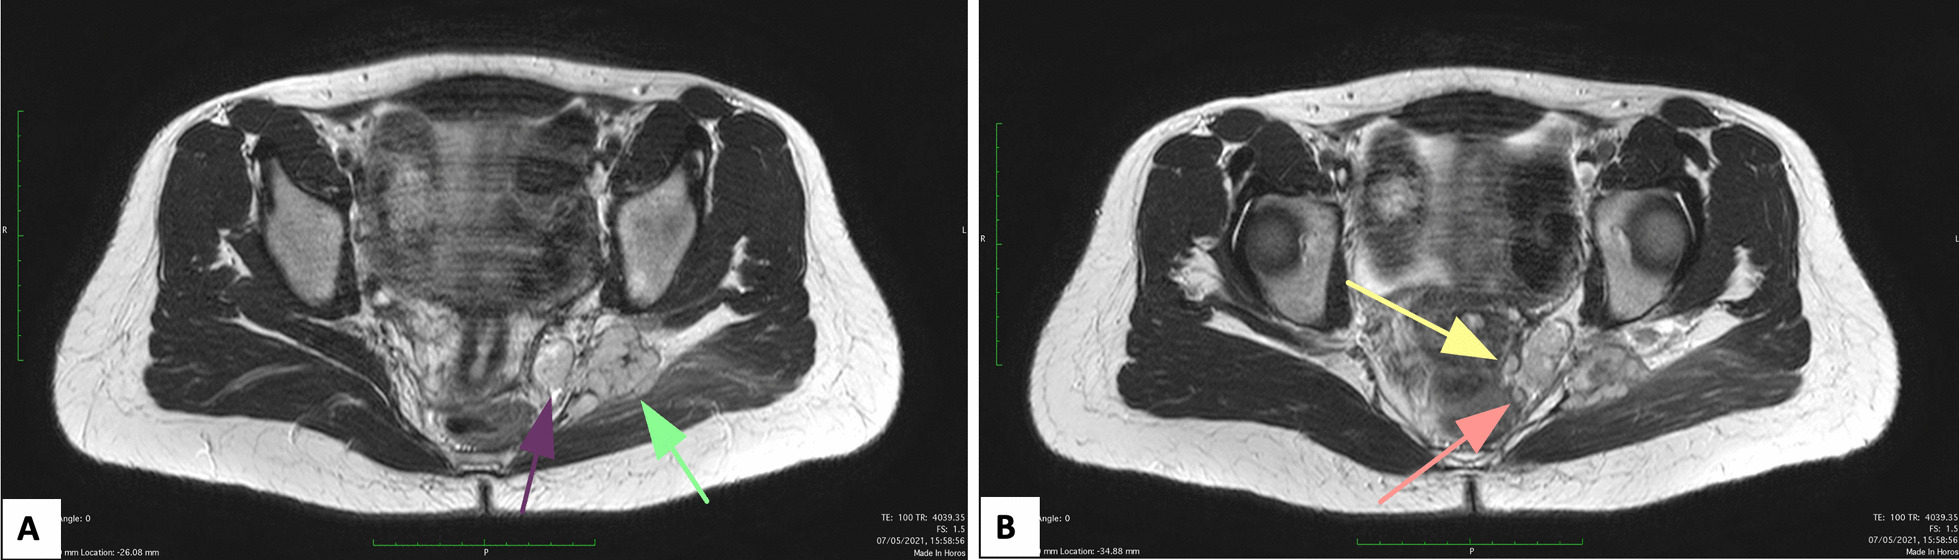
Magnetic Resonance Imaging (MRI) axial T1 weighted sequencies of the pelvis showing multiple nodules: A irregular nodule in gluteal region between the large gluteal muscle and the piriformis muscle (green arrow). Double nodule at the apex of ischiorectal fossa which is contiguous to the largest gluteal nodule (purple arrow); B two little nodules in the left obturator space, next to distal anal canal (yellow and pink arrow)
